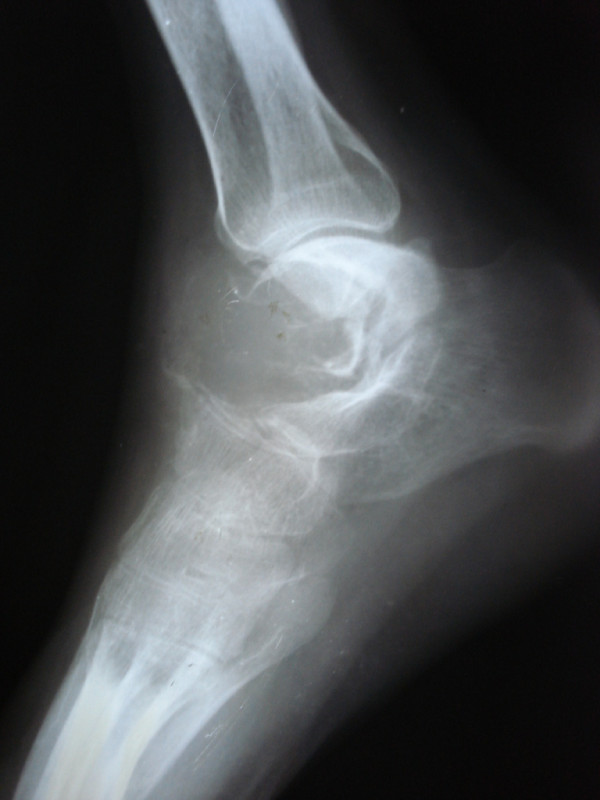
Lateral radiograph of ankle showing a radiolucent lesion occupying the talus with expansion and thinning of the cortex.
